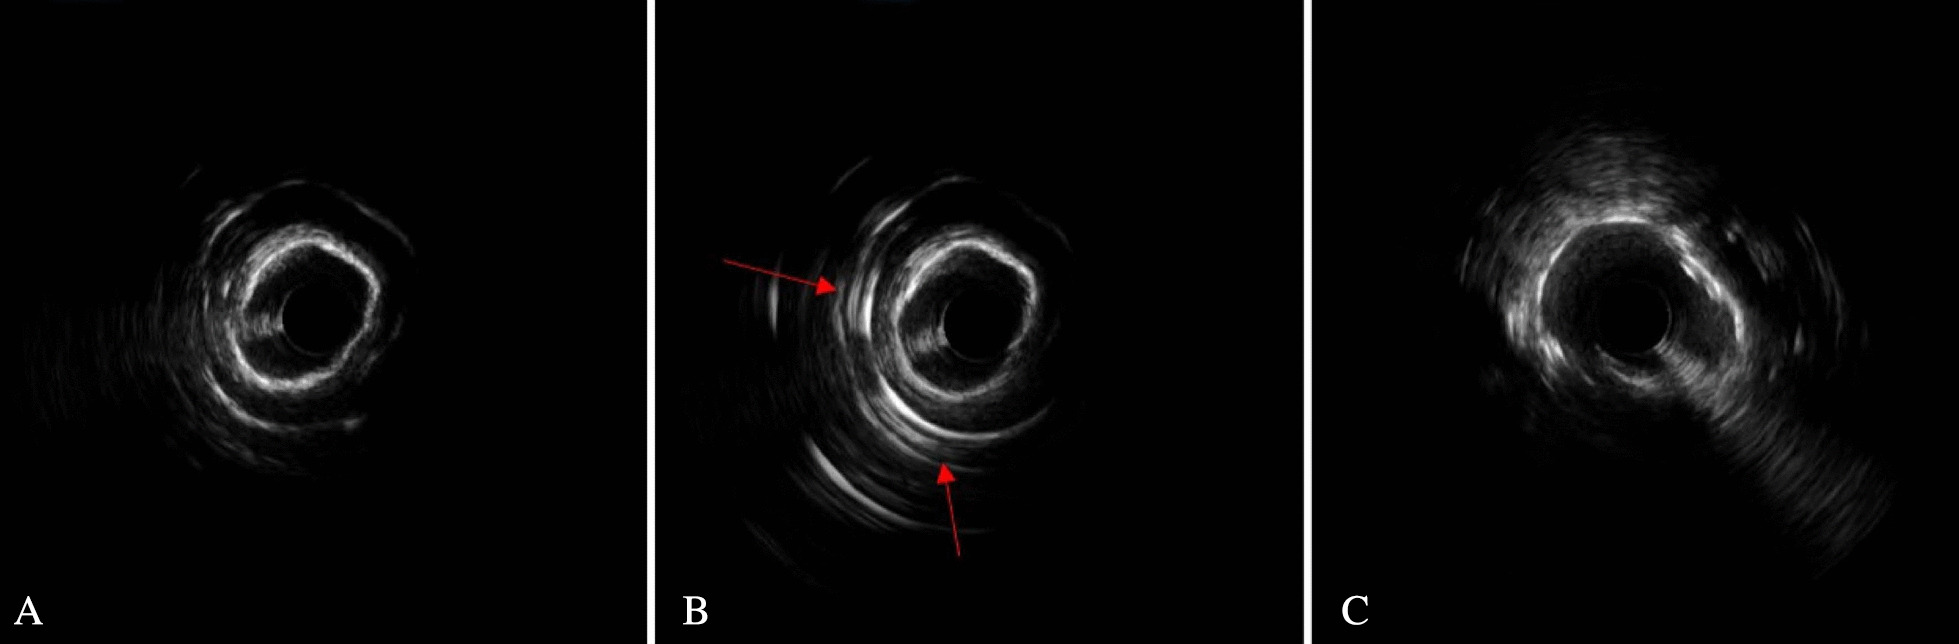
Representative one case of intravascular ultrasound (IVUS) guided rotational atherectomy (RA) images. A Pre-PCI IVUS images showed heavily calcified plaque with a 360° calcium arc. B After RA with a 1.5-mm burr, IVUS image showed reflection behind the calcification. The phenomenon usually was considered that the calcium was not thick. C After stent implantation with high pressure post-dilatation, IVUS images still showed stent under-expansion (73%)
